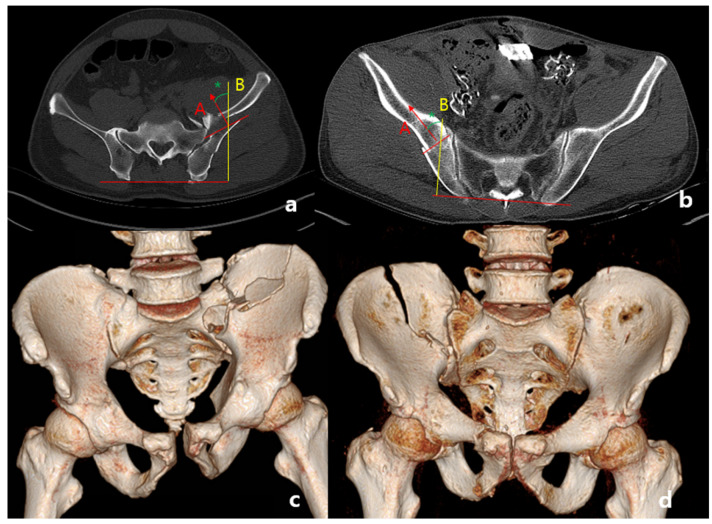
Comparing fracture lines of crescent fracture. Horizontal CT cut of ASFD (a) and LC (b). Three-dimensional reconstruction images of ASFD (c) and LC (d). * Angle between A and B is defined as crescent angle (green asterisk).
